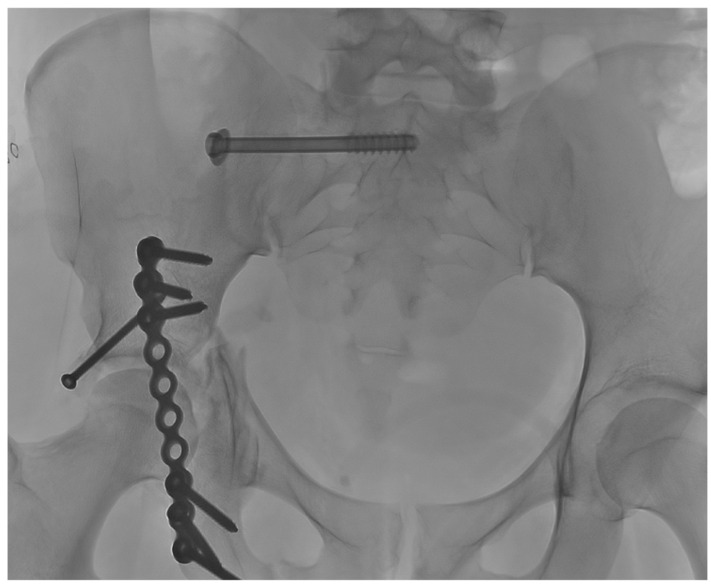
Postoperative X-ray shows the correct positioning of the screw.
